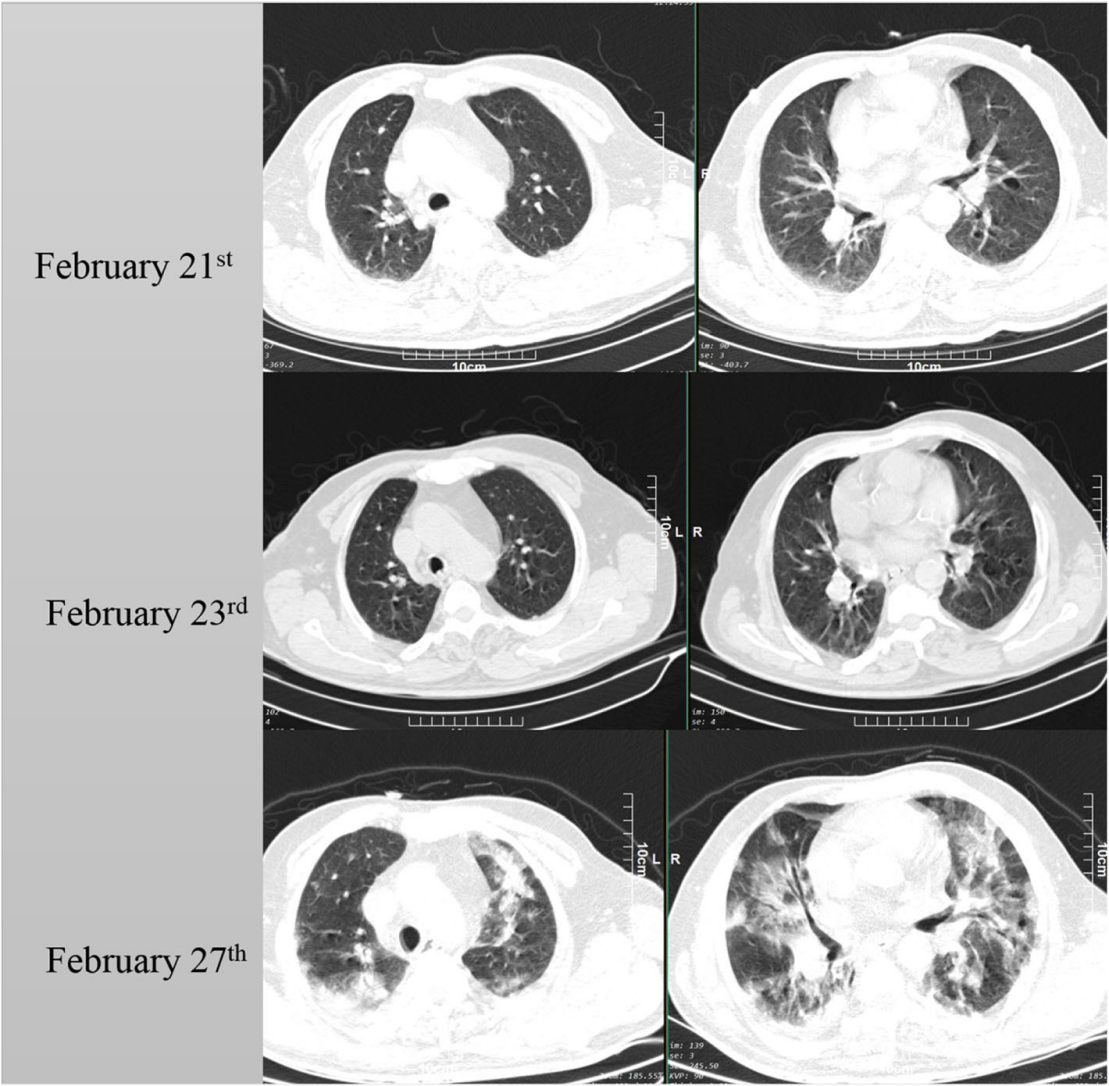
The first and second rows show the chest CT scan which was obtained at the emergency department, which shows no significant findings consistent with COVID19 except some linear atelectasis. The third row shows the chest CT on February 27 which has multifocal bilateral ground-glass opacities with some airspace consolidation suggestive of COVID19
